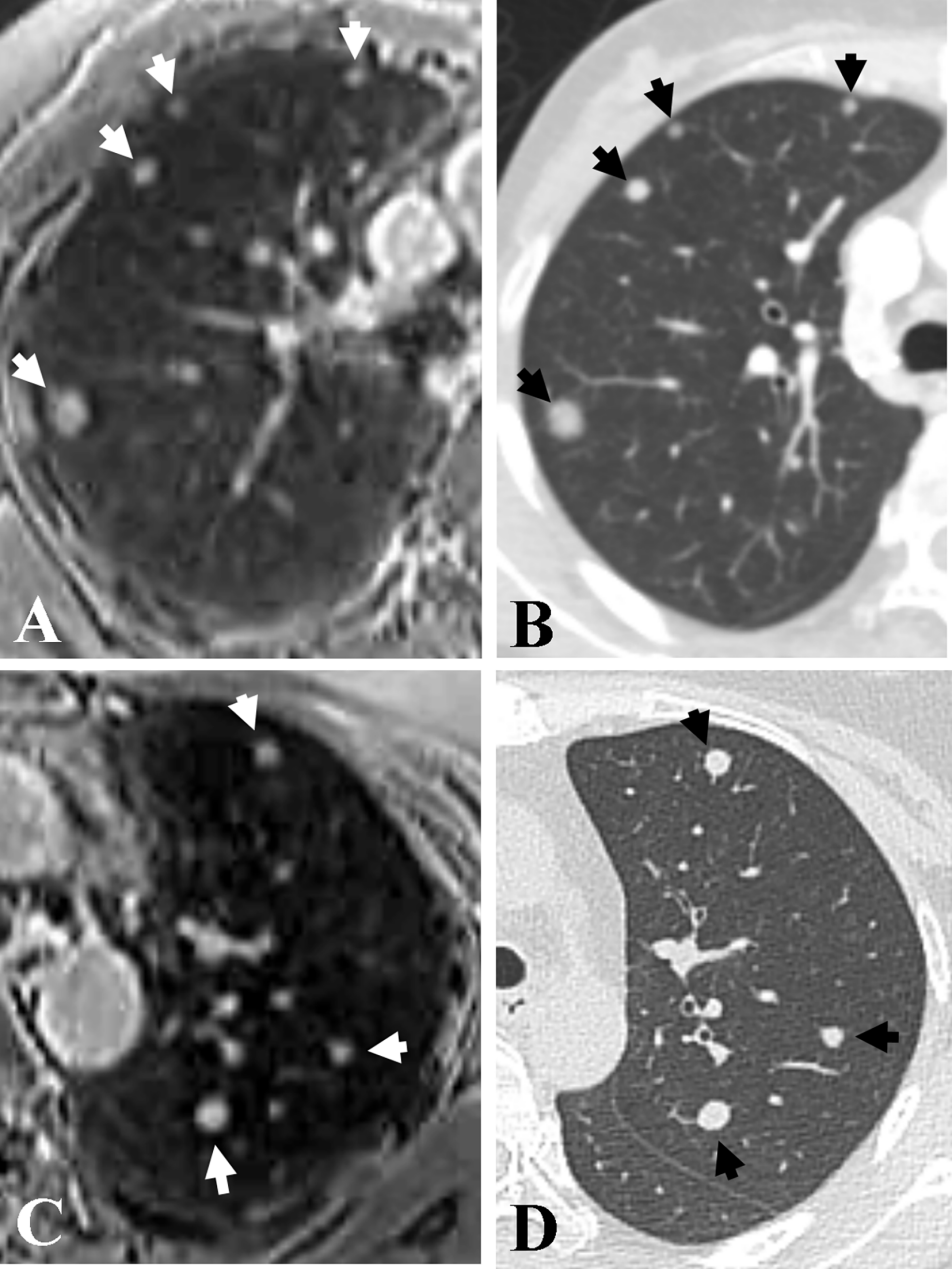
Representative axial images of accelerated spiral 3D UTE (A, C) and CT (B, D) for the detection of pulmonary metastasis. Seven nodules (arrows; range, 3.5 mm–10.6 mm in diameter) are detected in both upper lobes in a 38-year-old patient with bilateral breast cancer. The overall image quality rating for spiral 3D UTE images (A, C) was excellent.
